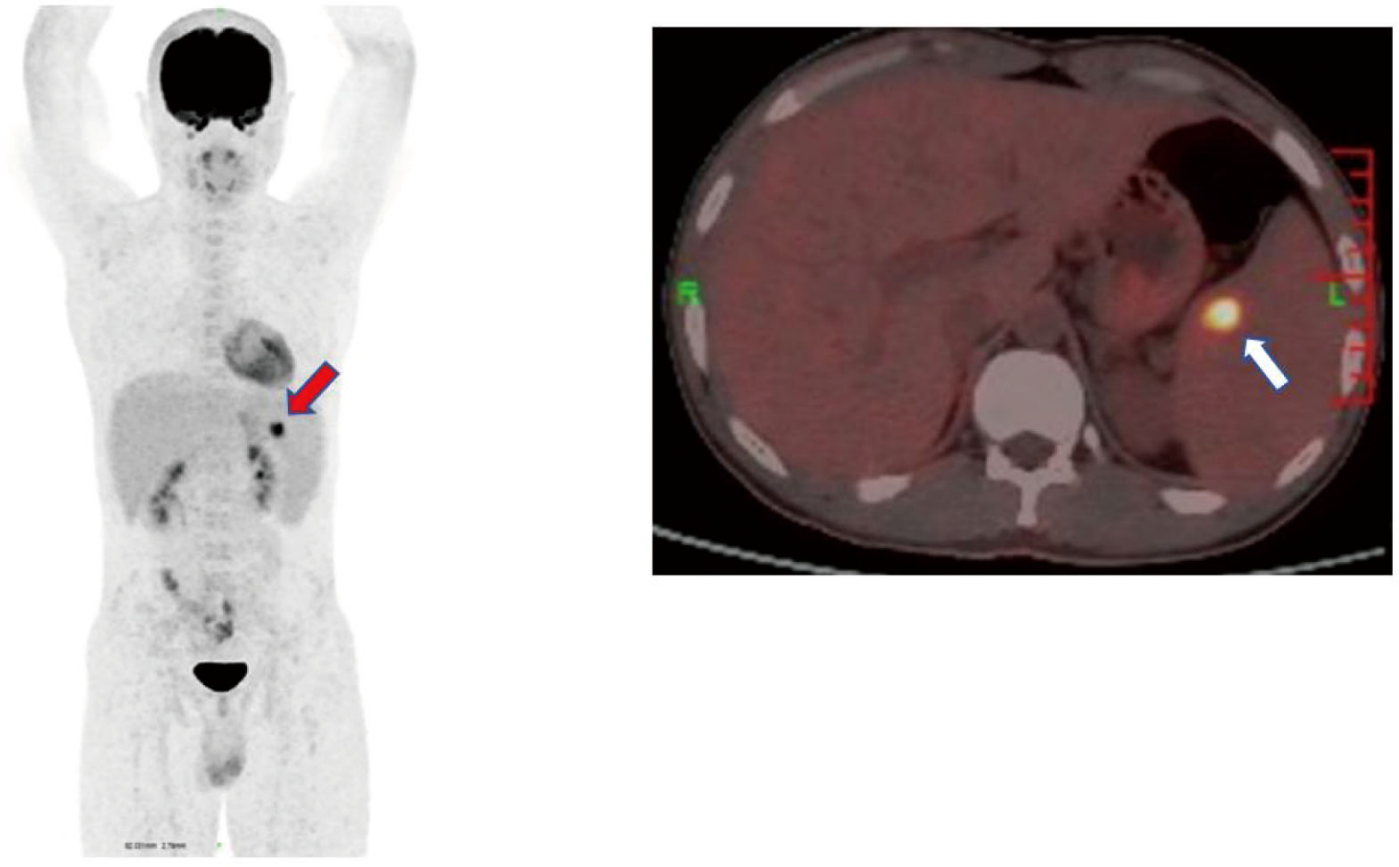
PET-CT whole-body imaging splenomegaly. There are focal hypermetabolic nodules inside, which cannot be ruled out as hemophagocytic changes. Both red and white arrows indicate spleens with hypermetabolic nodules. .
